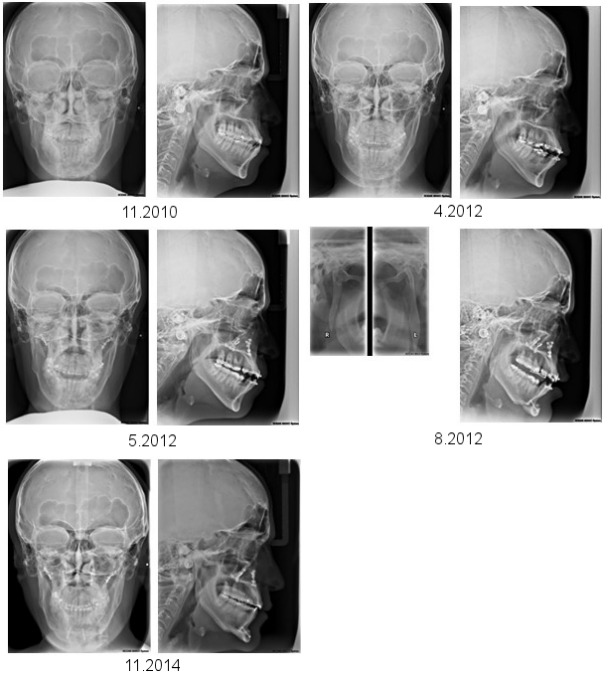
A series of cephalometric radiographs depicting the patient in anterior-posterior (left) or lateral projection (except the fourth series of radiographs). The first series shows the dental and skeletal relations prior to treatment. The second series shows the final position after completion of orthodontics prior to the first surgical procedure. The third series shows the position of the bones following maxillary osteotomy. The fourth series shows the vertical osteotomy of each mandibular ramus (left side), and the position of the jaws, including chin osteotomy, after the second surgical procedure. Finally, the fifth series demonstrates the relation of the jaws after completion of post-surgical orthodontics.
